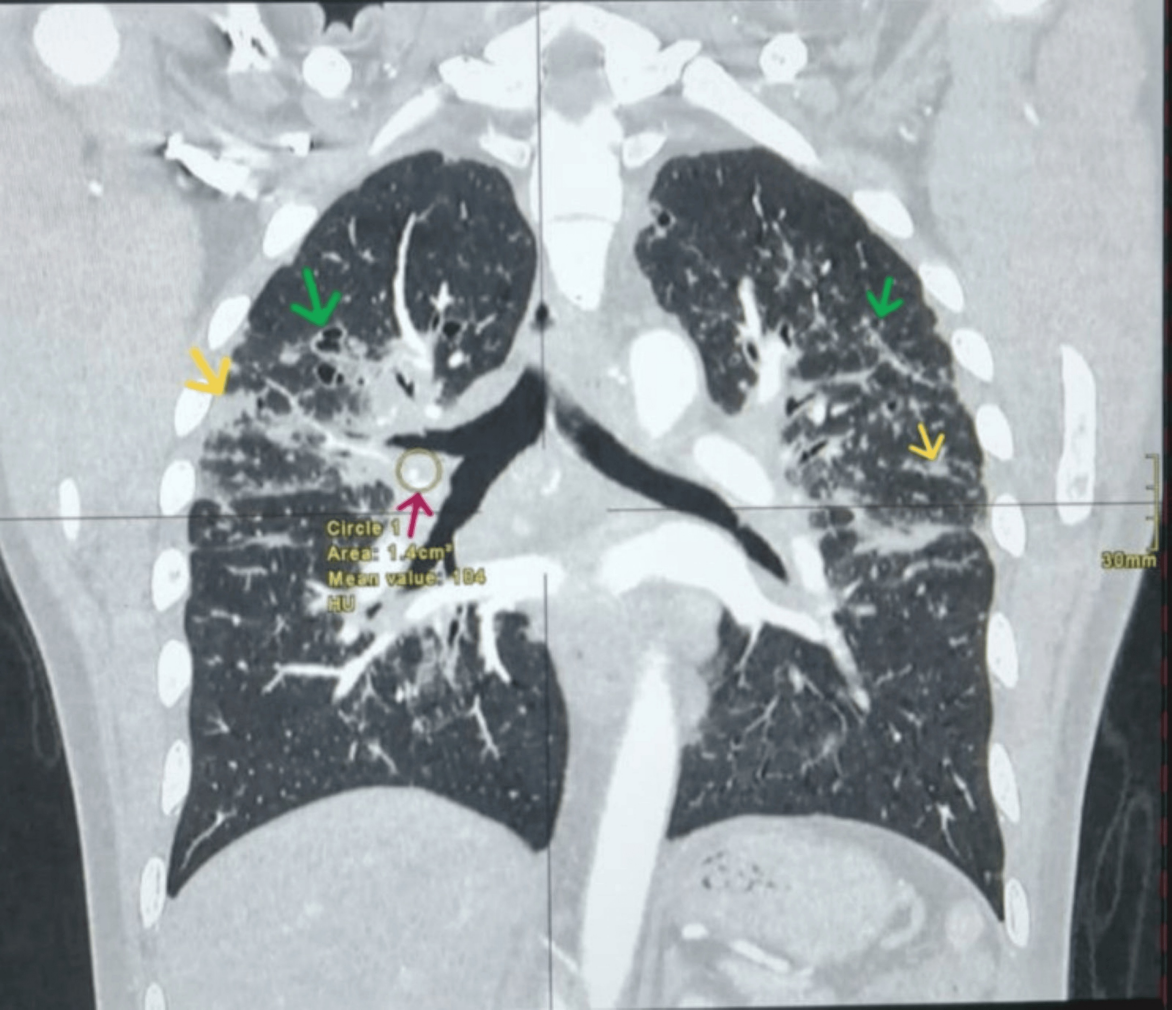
CT chest (coronal reconstruction, lung window, contrast-enhanced) demonstrating right hilar lymphadenopathy (red arrow), multiple bilateral subpleural nodules (yellow arrows), and cavitating pulmonary changes (green arrows).
